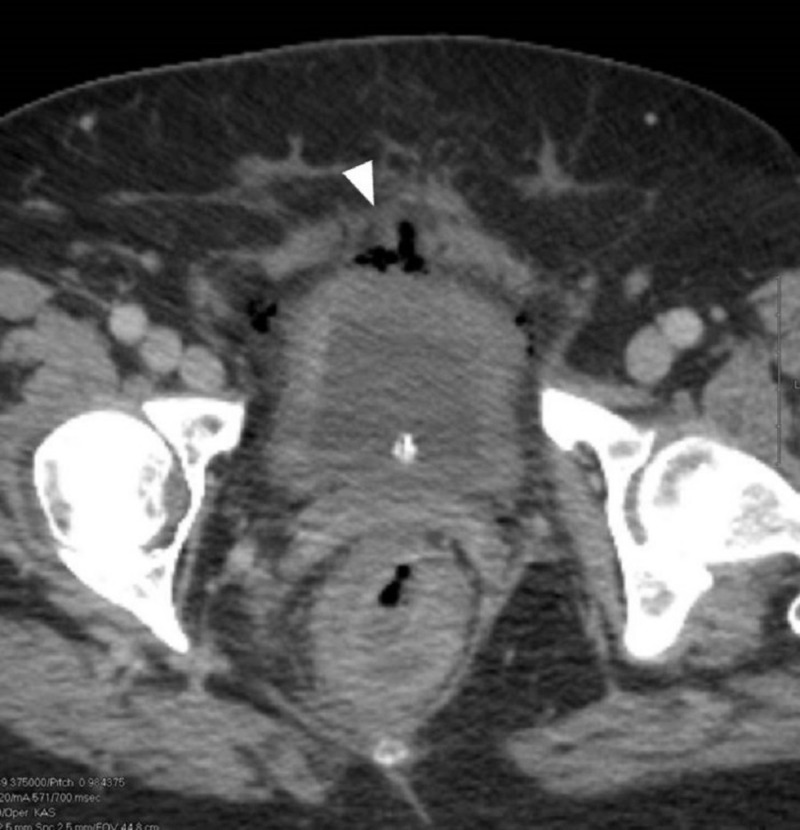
A computed tomographic (CT) scan after two days of therapy shows partial resolution of intra-mural gas from the urinary bladder walls
